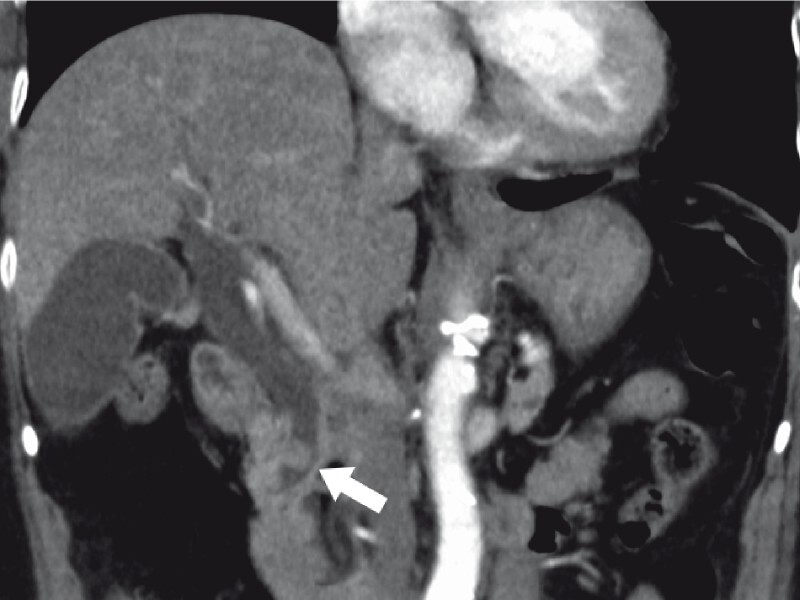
Computed tomography showing a common bile duct stone.
